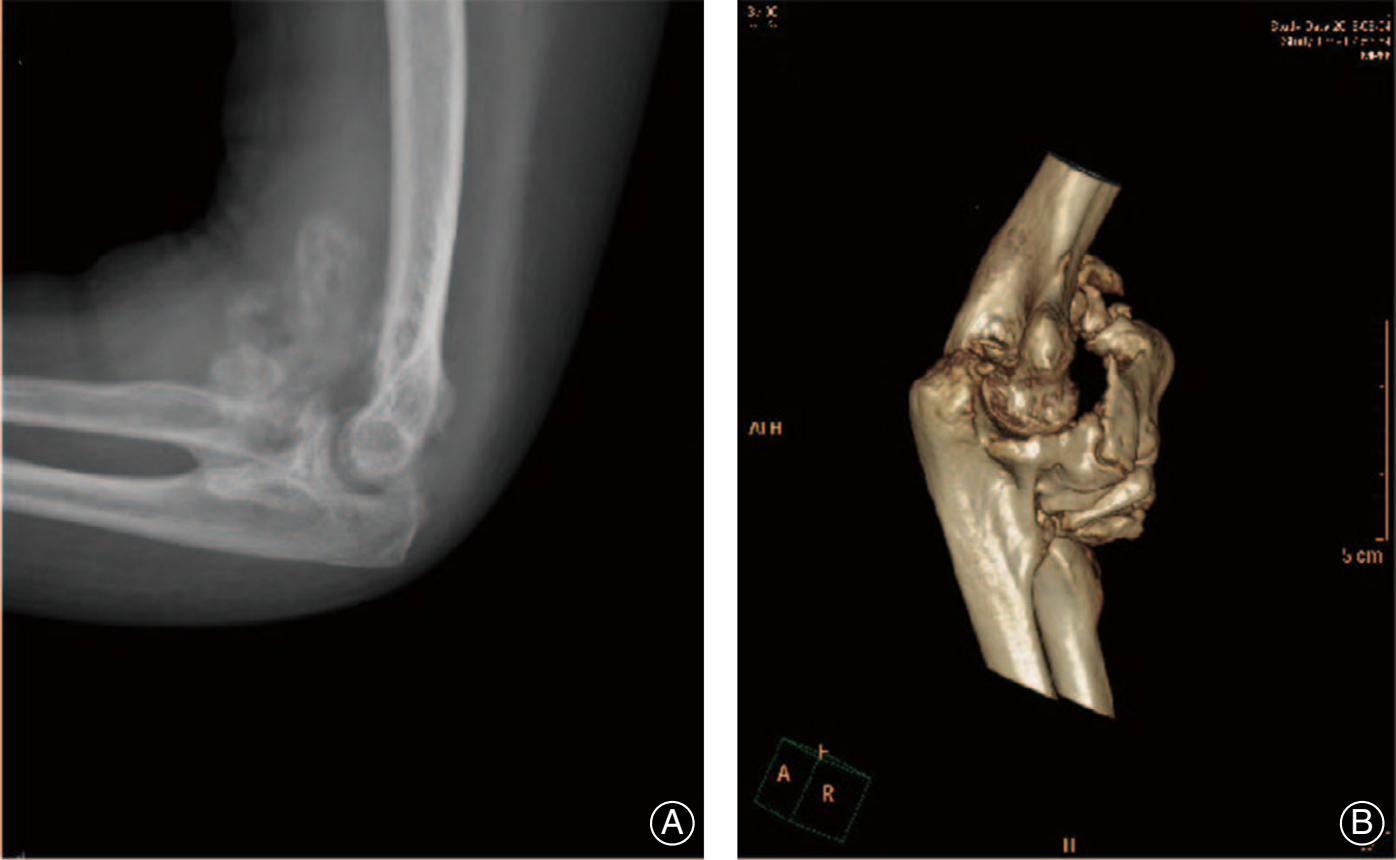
Heterotopic ossification (HO) formation after arthroscopic release. (A) X‐ray shows new HO formation at 5 weeks follow‐up. (B) CT scan shows mature HO at 8 months.
